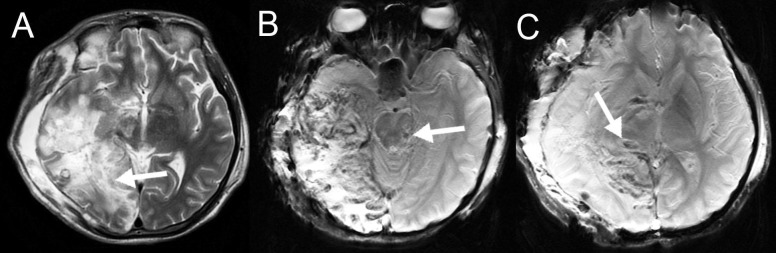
Case 1. Non-enhanced brain magnetic resonance imaging (MRI) after an emergent decompressive craniectomy. (A) Multiple ischemic changes in the right temporal lobe and in the right basal ganglia  areobserved (arrow). (B, C) Susceptibility-Weighted Magnetic Resonance Imaging show hypo-intense images in the mesencephalon and in the right anterior thalamus (arrows), demonstrating grade III diffuse axonal injury.
